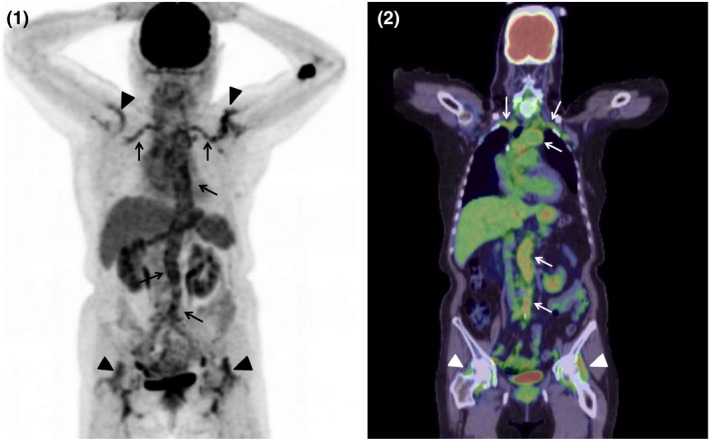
F‐18 FDG‐PET/CT showed increased FDG uptake in the wall of aorta, bilateral subclavian arteries (1 and 2, arrows), and shoulders and hip joints (1 and 2, arrowheads).
